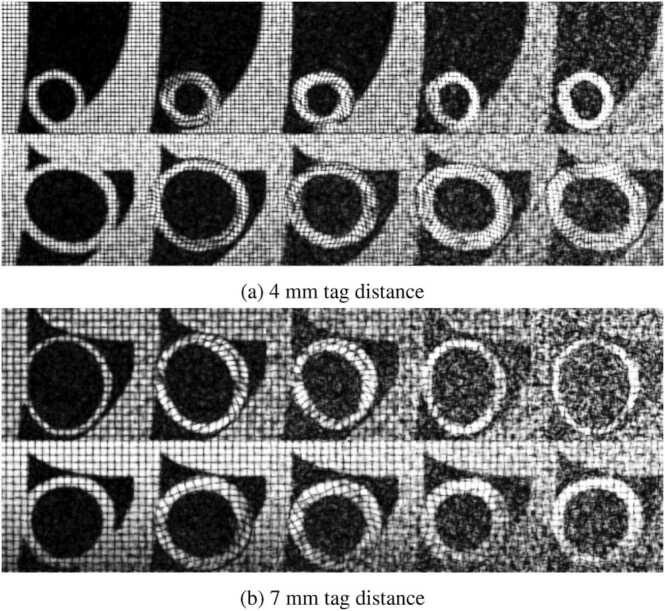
Examples of synthetic 3D tagged MRI data with 4 mm (top panel) and 7 mm (bottom panel) tag distance. Each row displays the tagged pattern on a mid-ventricular slice for selected cardiac phases. *3D* three-dimensional, *MRI* magnetic resonance imaging.
